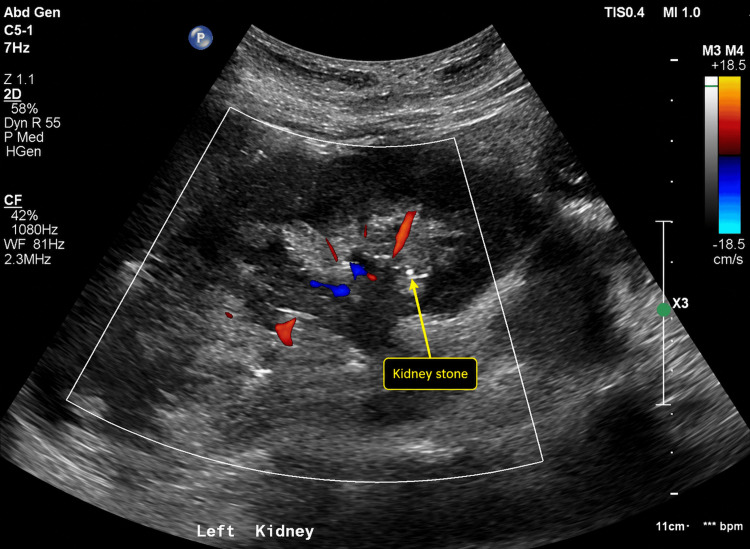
Ultrasound of the abdomen Ultrasound of the left kidney demonstrating a small non-obstructive renal calculus (arrow) without hydronephrosis or significant obstructive uropathy. The finding was considered incidental and insufficient to explain the recurrent severe neurovisceral attacks.
